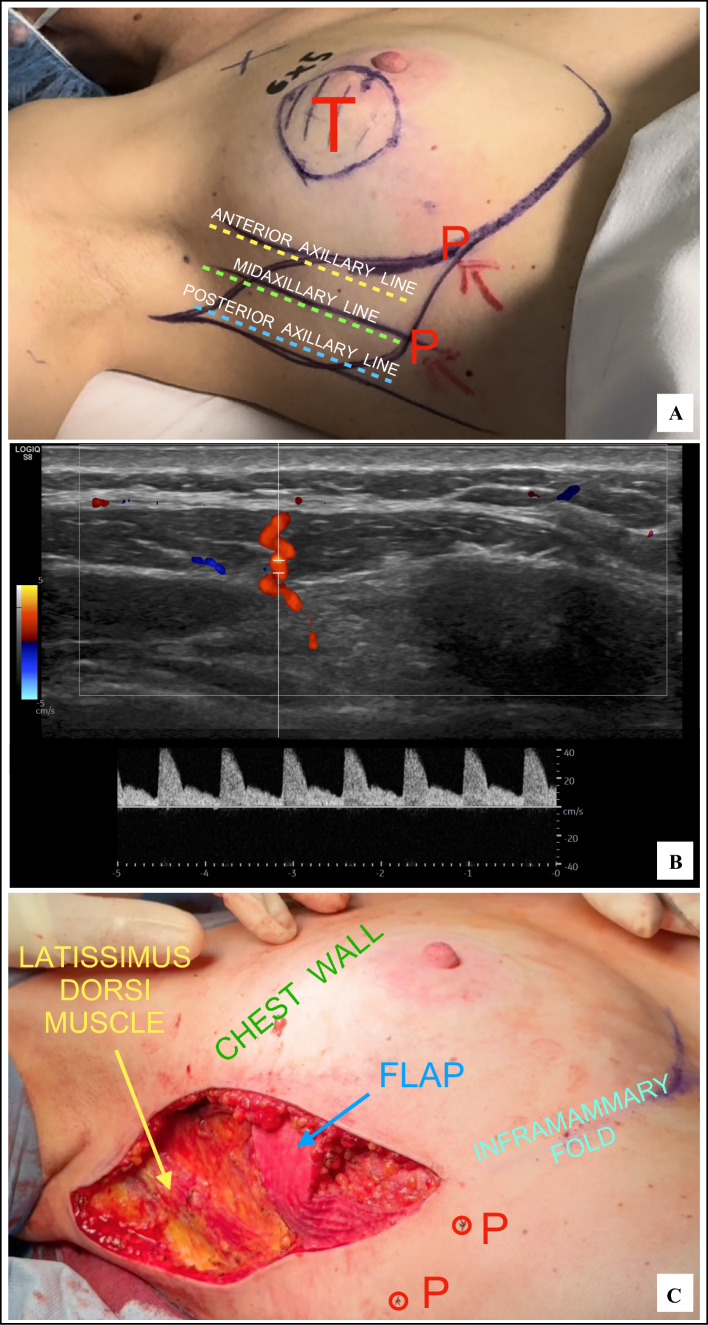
A Preoperative planning of an LICAP flap based on the vascular anatomy of chest wall perforators. B Power Doppler ultrasound image showing a LICAP pedicle with ideal peak systolic velocity. C Anatomic landmarks after flap movement in the final position. LICAP, lateral intercostal artery perforator; T, tumor; P, perforator
